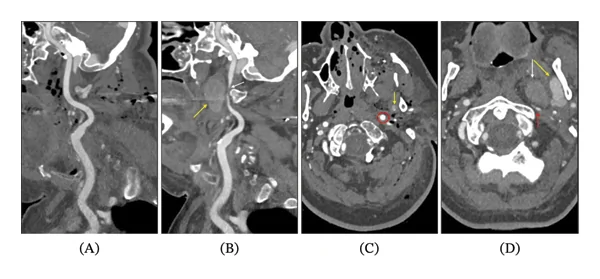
A CT angiogram of 75‐year‐old patient who presented to the hospital for a gunshot wound to the left side of the face. Maximum intensity projection reconstruction of the left internal carotid artery at Day 0 (A) and Day 14 (B) shows a short segment high‐grade or near‐complete occlusion of the distal cervical left internal carotid artery (white arrow), with distal reconstitution and normal vessel caliber. The yellow arrow points to pseudoaneurysm of the internal mammillary artery branch of the external carotid artery. (C) Axial CT angiogram of the patient’s neck at Day 0. The yellow arrow shows wispy contrast enhancement in the proximal portion of the left internal maxillary artery suggestive of a small pseudoaneurysm or subtle extravasation. The red circle shows widely patent internal carotid artery. (D) Axial CT angiogram of the patient’s neck at Day 14. The red arrow shows a short segment high‐grade or near‐complete occlusion of the distal cervical left internal carotid artery. The yellow arrow demonstrates arterial hyperenhancement, and the white arrow shows venous phase enhancement concerning for venous and arterial pseudoaneurysms from penetrating injury.
